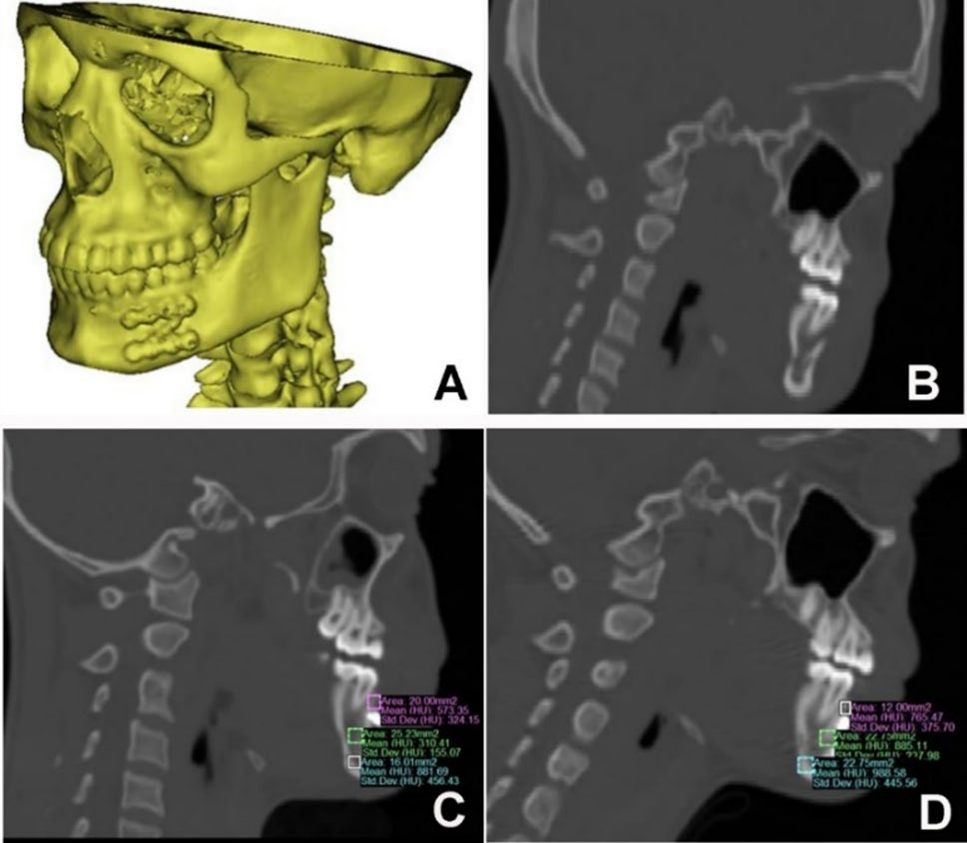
(Study case): A 3D reconstruction of mandible treated with customized z miniplate, B preoperative CT, C measuring bone density at immediate postoperative CT, D measuring bone density after 6 months follow up CT
